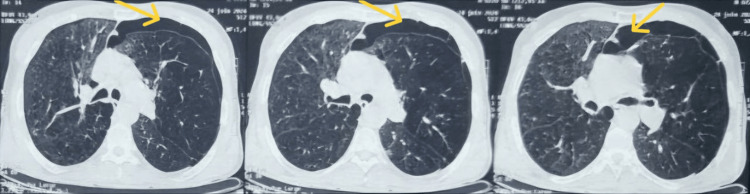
Computed tomography before chest tube insertion The admission chest computed tomography scan revealed an anterior left-sided pneumothorax (indicated by yellow arrows)
